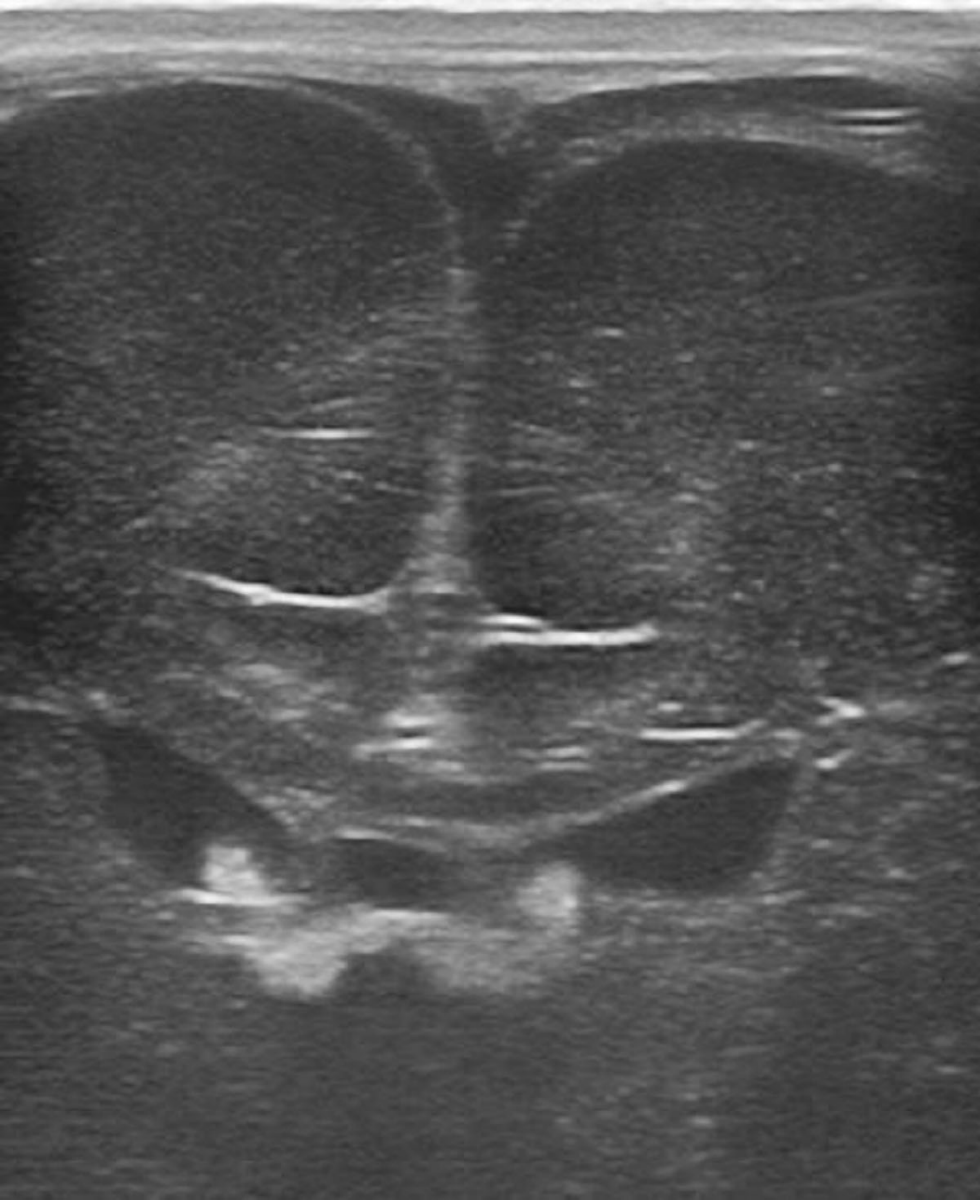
Infant with agyria (complete lissencephaly), the most serious form of abnormal neuronal migration. The coronal sonogram shows the complete absence of convolutions.
